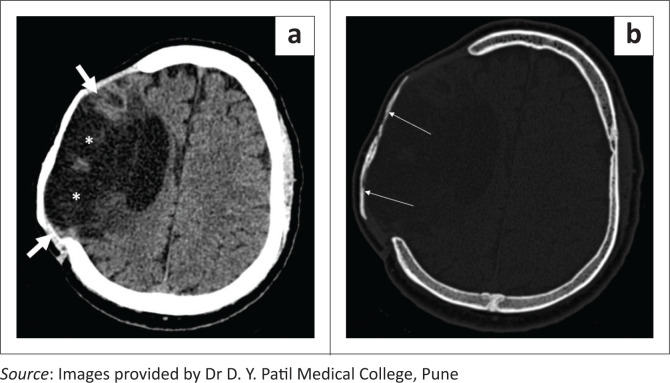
Axial soft tissue (a) and bone window (b) CT brain images in a patient with a right fronto-parietal decompressive craniotomy (a, thick white arrows) demonstrate extracranial herniation of brain tissue (a, asterisks) with thinning and remodeling of the bone flap (b, thin arrows).
